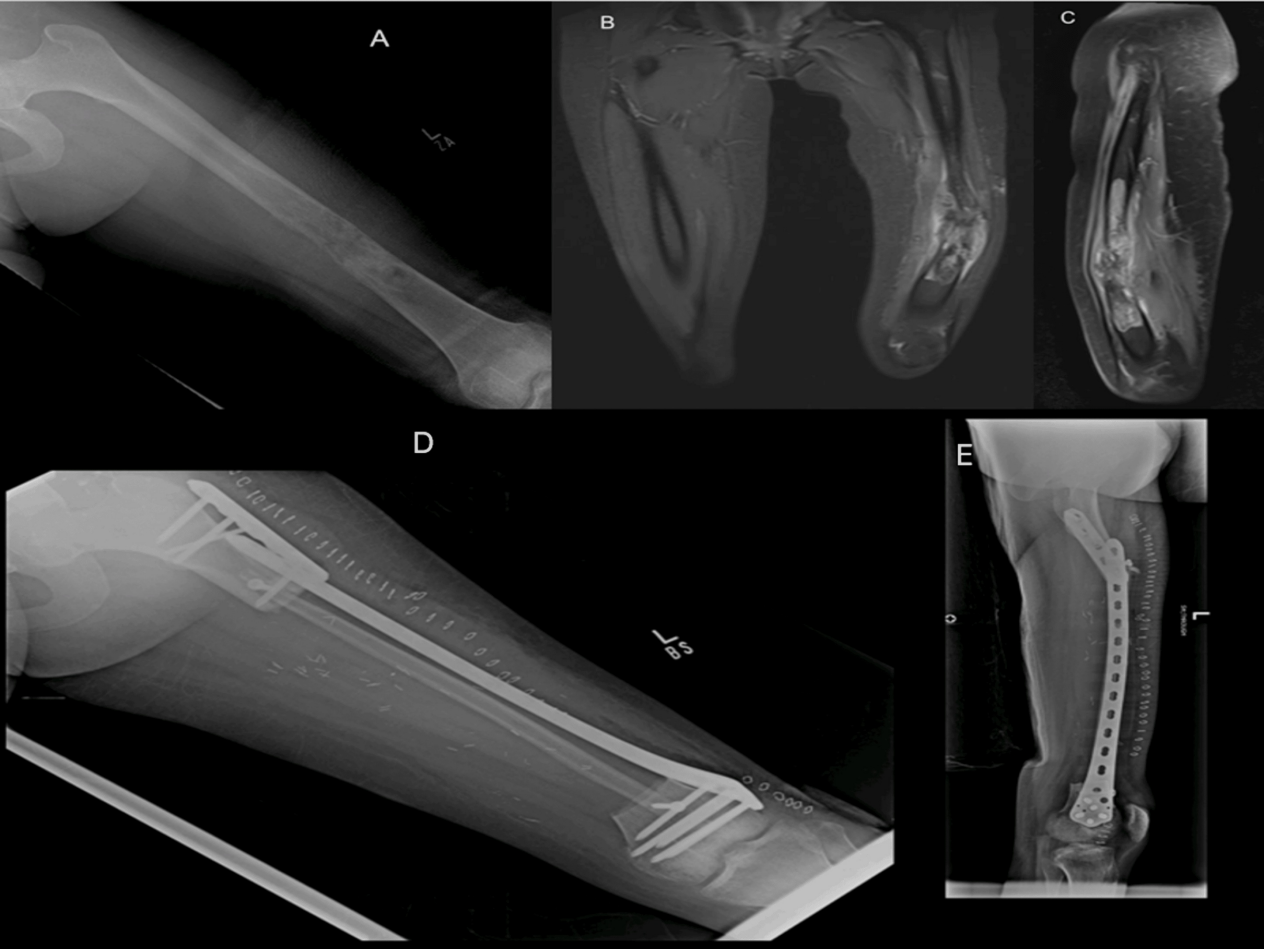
Diagnostic, surgical, and post-treatment imaging of a 13-year-old patient with Osteosarcoma of the left femur A 13-year-old girl presented with a two-year history of progressively worsening left leg pain and weight loss without neurological symptoms. A biopsy of her left femur confirmed the diagnosis of osteosarcoma. Following this diagnosis, she underwent neo-adjuvant chemotherapy according to the MAP protocol for two cycles. Subsequently, she underwent surgery involving excision of the tumor, vascularized free fibula grafting, and placement of a distal femur plate. • Figures A-C: Post-chemotherapy MRI images showing the response to treatment and planning for surgical intervention. • Figure D-E: Post-operative X-rays demonstrating the placement of the distal femur plate and the vascularized free fibula graft.
